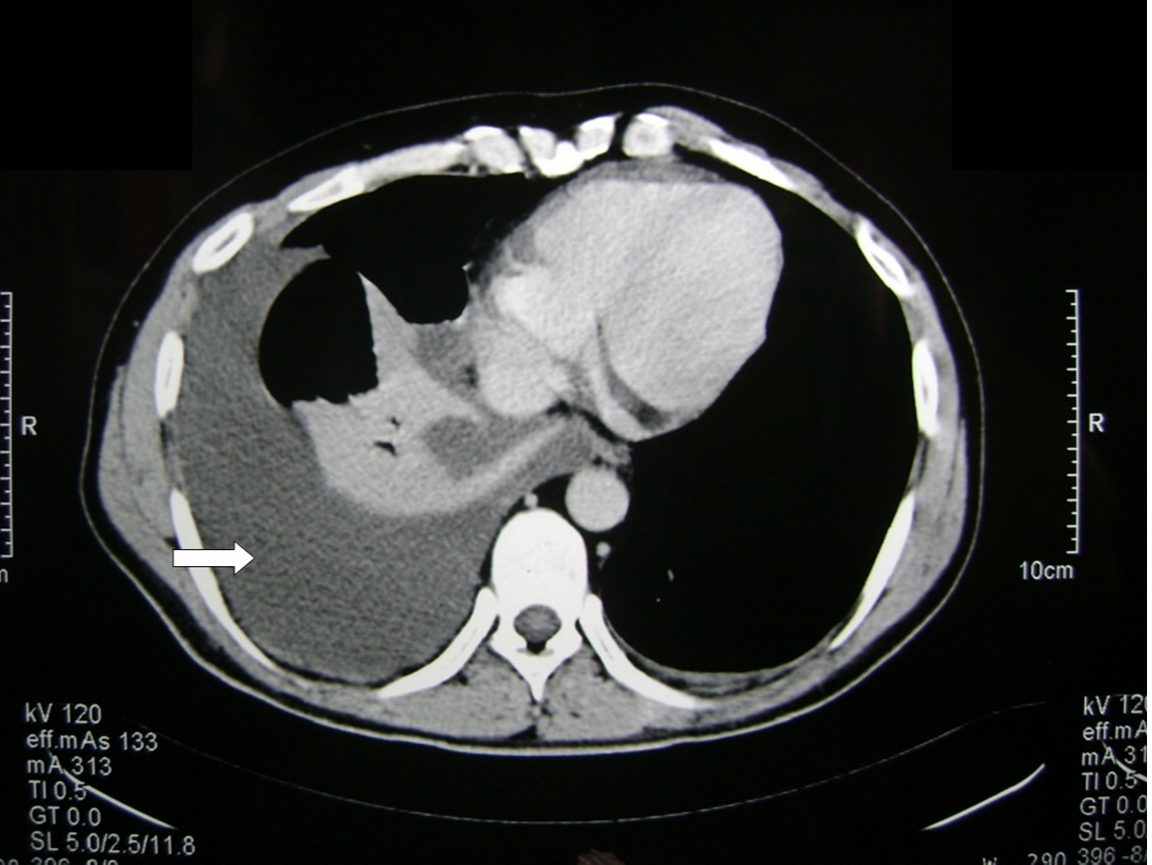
Contrast-enhanced computed tomography of the chest revealing pleural fluid accumulation in both pleural cavities. The occurrence of lung parenchyma is normal.
